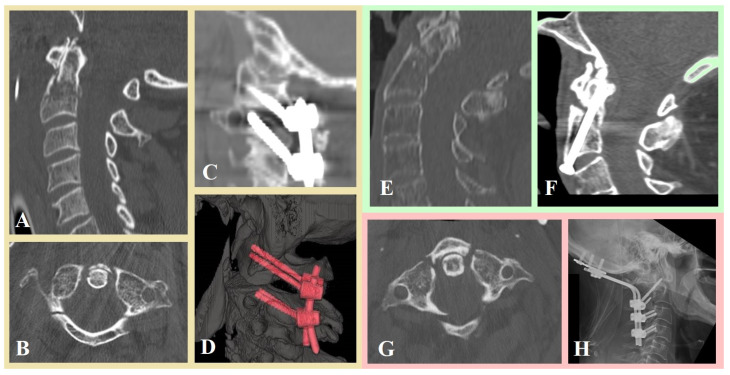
Preoperative cervical CT scans (A,B) of an 81-year-old man with C1 and C2 fractures underwent C1–C2 fixation according to Harms technique (C,D). Preoperative cervical CT scan (E) of an 83-year-old man with an odontoid C2 fracture type II according to Anderson and D’Alonzo classification underwent C2 dens screwing (F). Preoperative cervical CT scan (G) of an 82-year-old woman with a Jefferson type III C1 fracture treated surgically with occipitocervical fixation (H).
